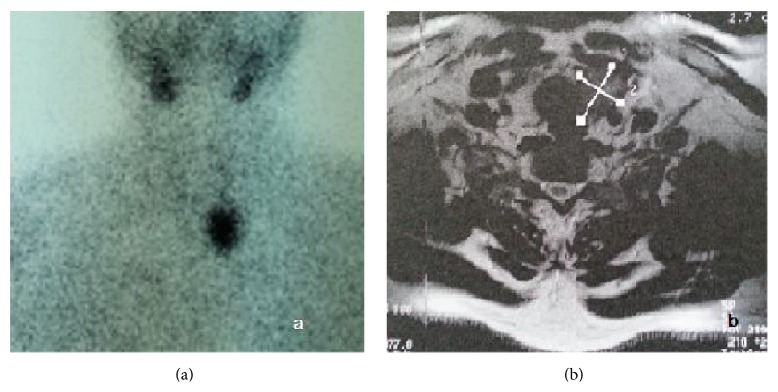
A 45-year-old man with parathyroid carcinoma: (a) Technetium-99m Sestamibi scan and (b) Magnetic Resonance Imaging scan showing the parathyroid tumor.
